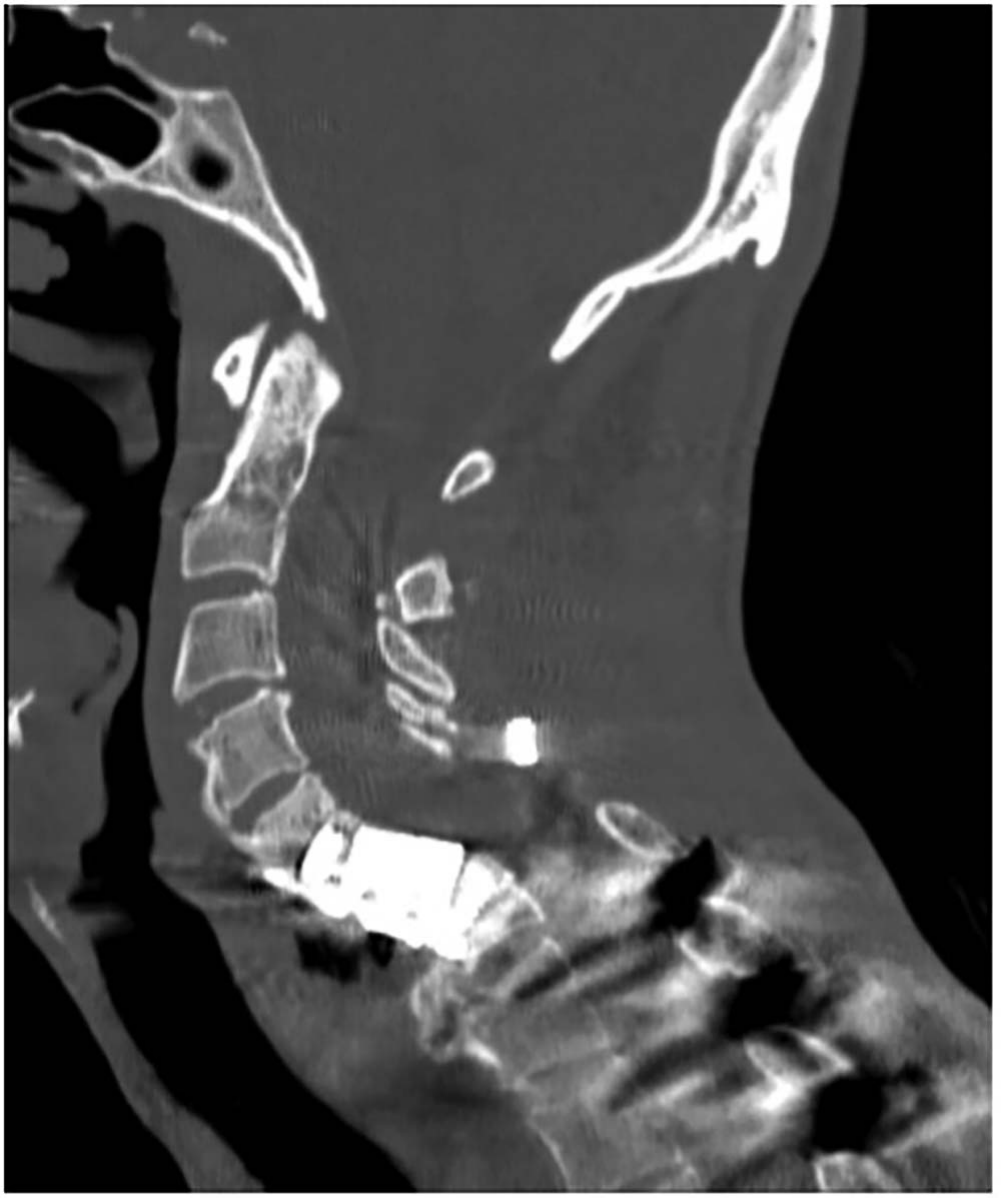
Initial sagittal CT without contrast after patient presented with symptoms of esophageal injury demonstrating increased gas density at the site of C6 corpectomy and post cricoid region.
